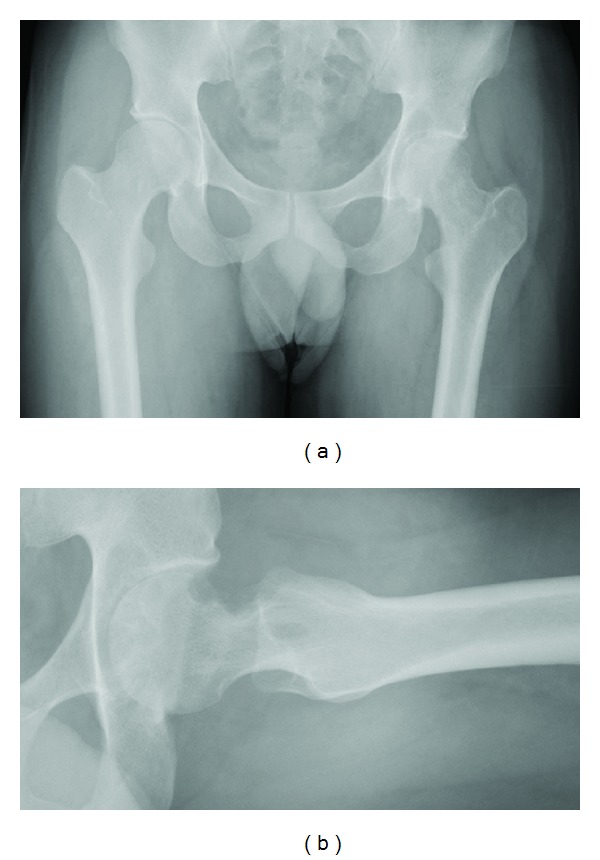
(a, b) Joint narrowing and multiple bone erosions of the hip joint are seen on this roentgenogram.
